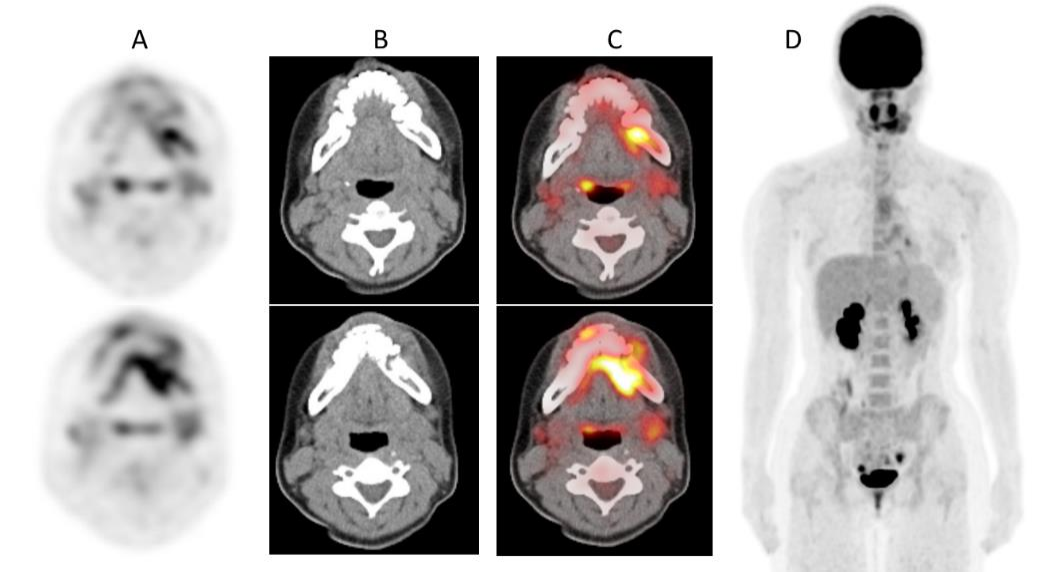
Transverse ^18^F-FDG PET (A), CT (B), and PET/CT fused (C) images show increased uptake in the tumor (SUV_max_=7.79). ^18^F-FDG PET maximum intensity projection image (D) reveals no other abnormal uptake suggestive of metastasis
